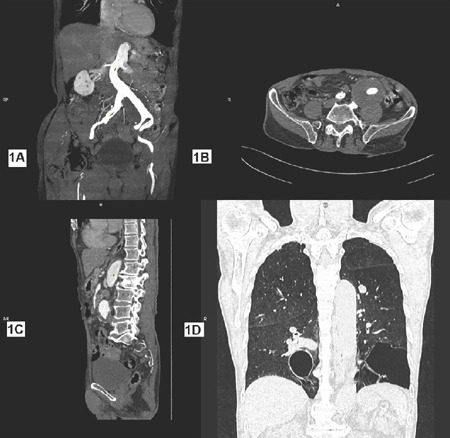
1A) Computed tomography (CT) angiogram coronal section shows large
